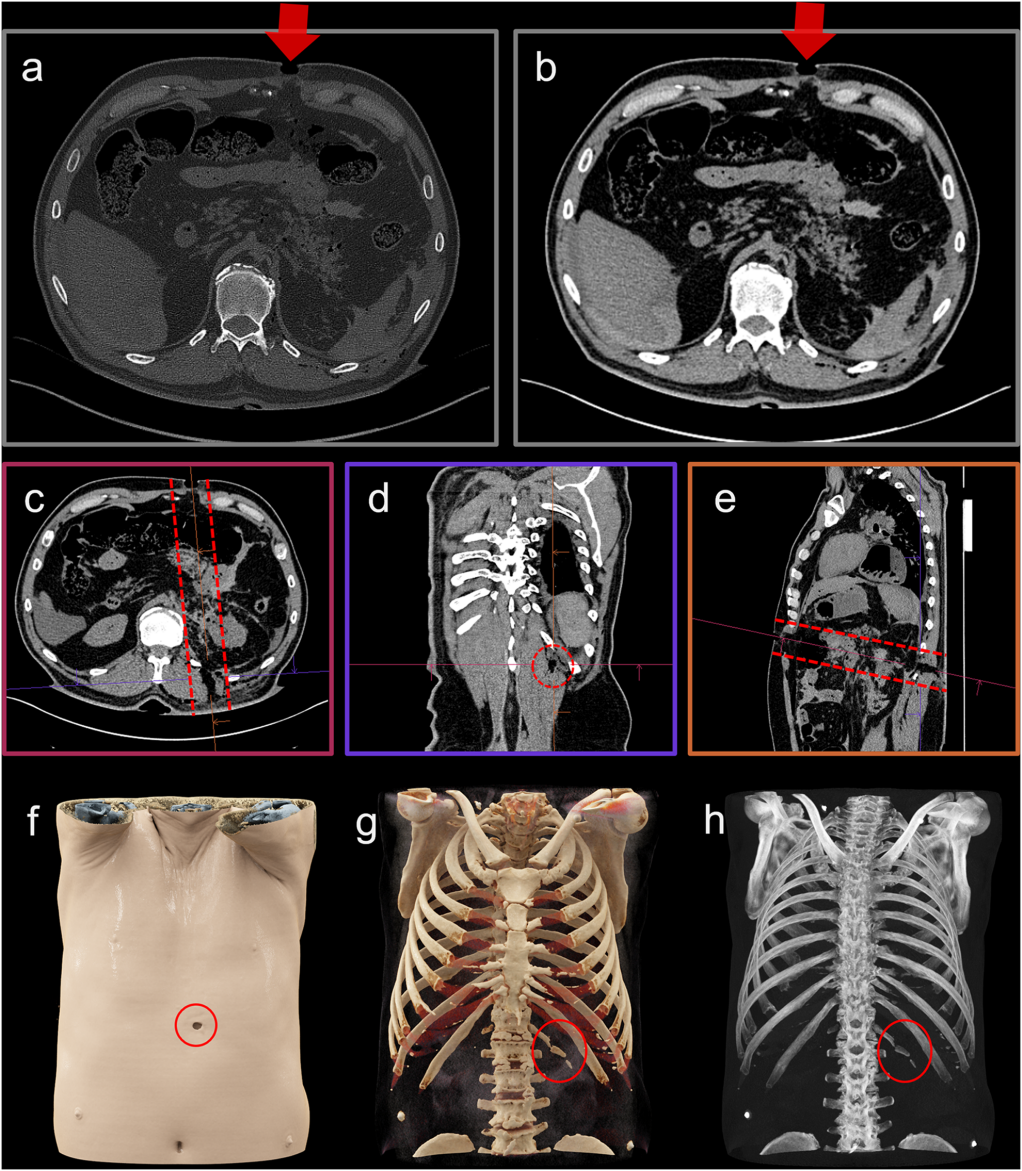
Case example depicting an abdominal gunshot wound (51). The axial slices with a hard kernel and bone window (a) and a soft kernel with soft tissue window (b) represent the two standard CT data visualizations. In these images, which display slices perpendicular to the scan axis, only the entry wound is visible (a and b, arrow). One advantage of virtual autopsy using CT is its ability to provide three-dimensional data, and through multi-planar reformations (c–e), the orientation of key findings—such as the bullet trajectory [(c–e), dashed markings]—can be adjusted accordingly. Three-dimensional visualizations, including volume rendering (f), cinematic rendering (g), and maximum-intensity projection (h), offer impressive depictions that enhance understanding, even for non-radiologists. For example, the gunshot wound at the skin surface is clearly visible [(f), red circle], as is a fracture of the 13th rib [(g,h), red circle]. Different visualization techniques can be selected depending on the specific findings; but whether those findings are recognized depends on the observer's training and experience.
